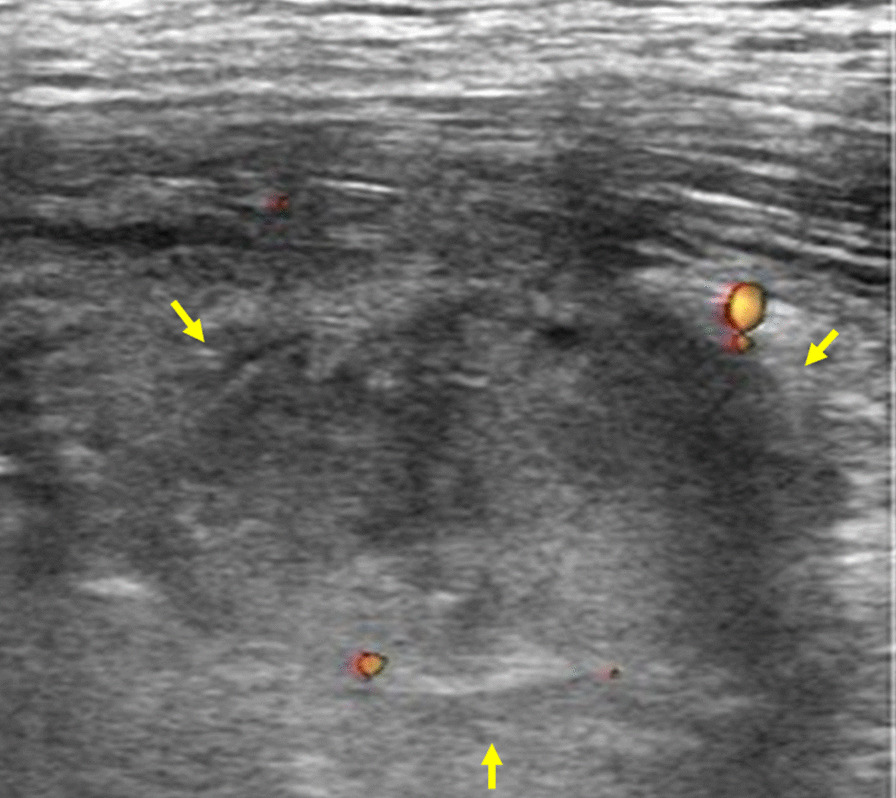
Ultrasonography of left erector spinae muscle showed an iso-hypoechoic tumor 30 mm in size without blood flow (yellow arrow).
